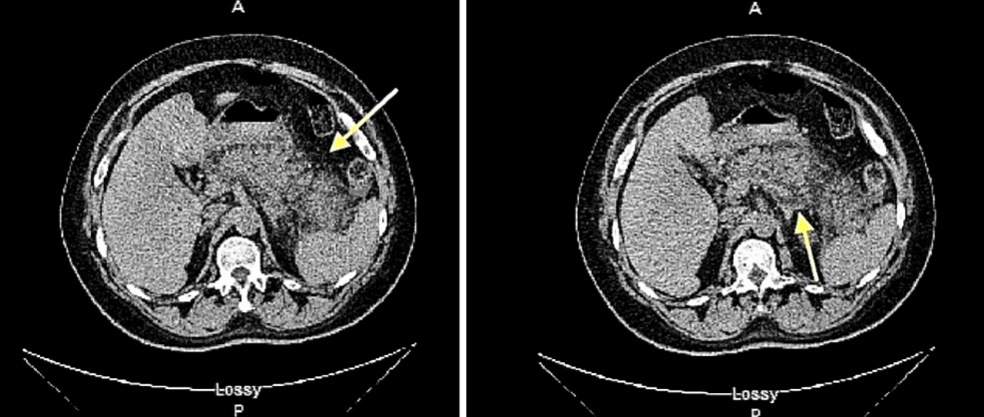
Abdominal CT scan Abdominal CT depicting an enlarged pancreas, significant peripancreatic edema/stranding (yellow arrow), and peripancreatic fluid extending to the left pararenal space and paracolic gutter, all of which are classic signs of pancreatitis.
